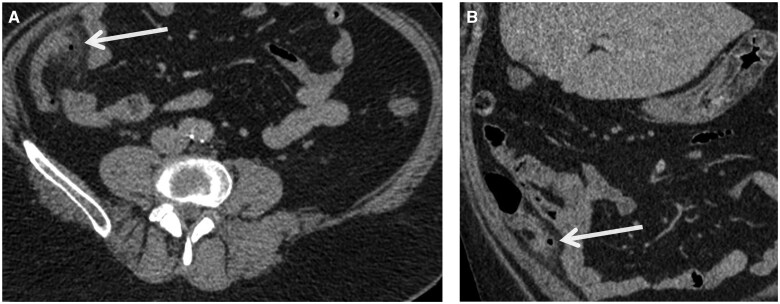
Contrast-enhanced CT of the abdomen in portal venous phase. (A) Axial section. (B) Coronal section. At the medial aspect of the appendix (arrows), there are several diverticula that are markedly inflamed and surrounded by fat stranding, consistent with appendiceal diverticulitis.
